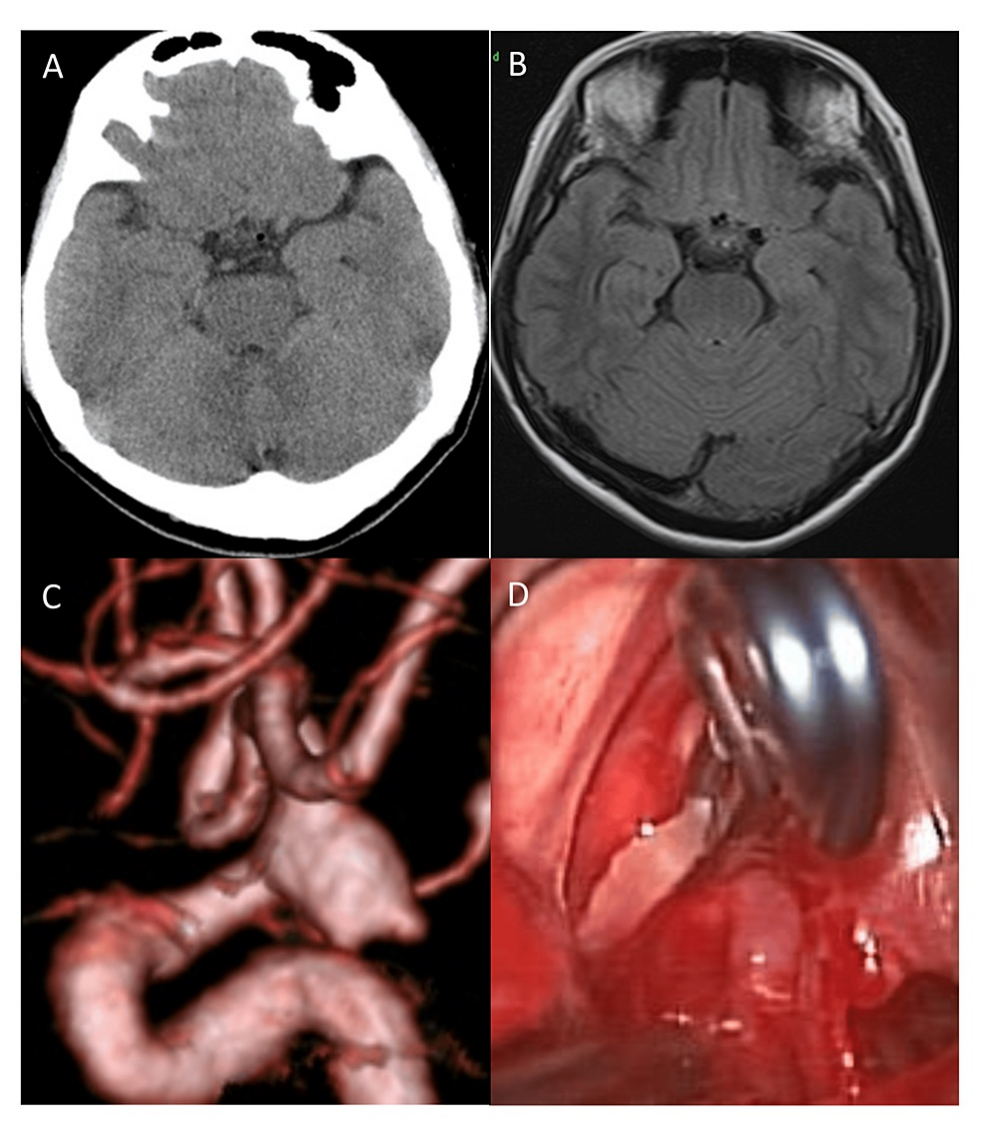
Case 2 CT (2A) and FLAIR-MRI (2B) scans at the initial examination. No SAH was detected. Computed tomographic angiography (CTA) revealed the inferolateral-directed ICA-PcomA aneurysm with bleb (2C). Intraoperative findings (2D) revealed that the aneurysm firmly adhered at a site behind the anterior clinoid ligament. FLAIR: fluid-attenuated inversion recovery; SAH: subarachnoid hemorrhage
